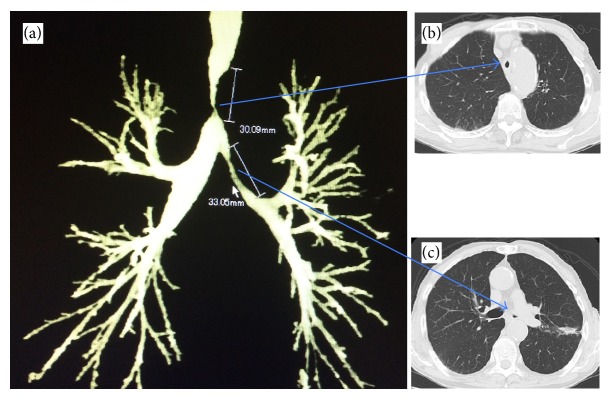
(a) Three-dimensional chest computed tomography (CT) showing stenoses of distal trachea and left main bronchus. (b) Stenosis of distal trachea. (c) Stenosis of left main bronchus.
